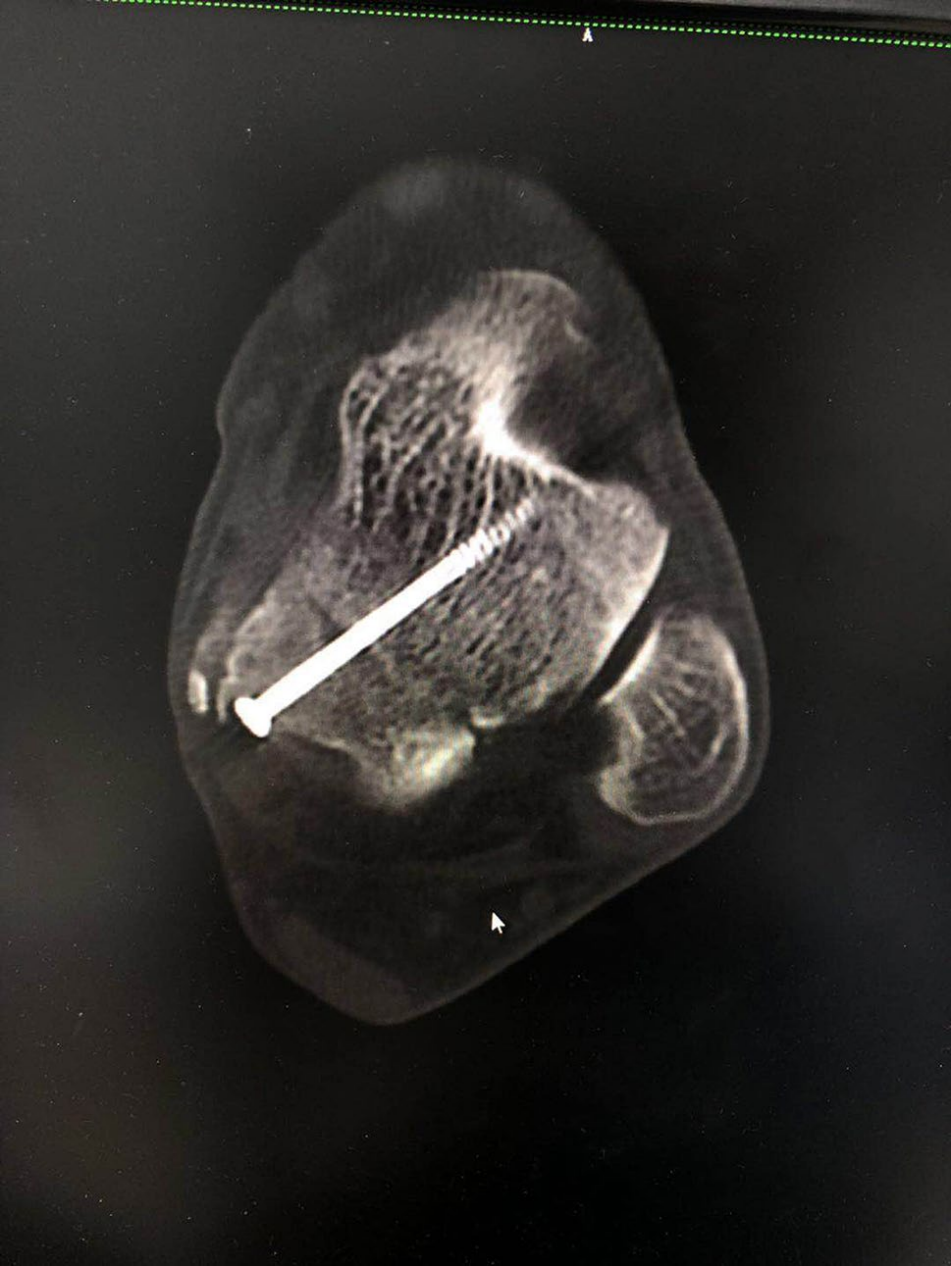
Axial view of CT scans showing that the internal fixation was performed properly.
